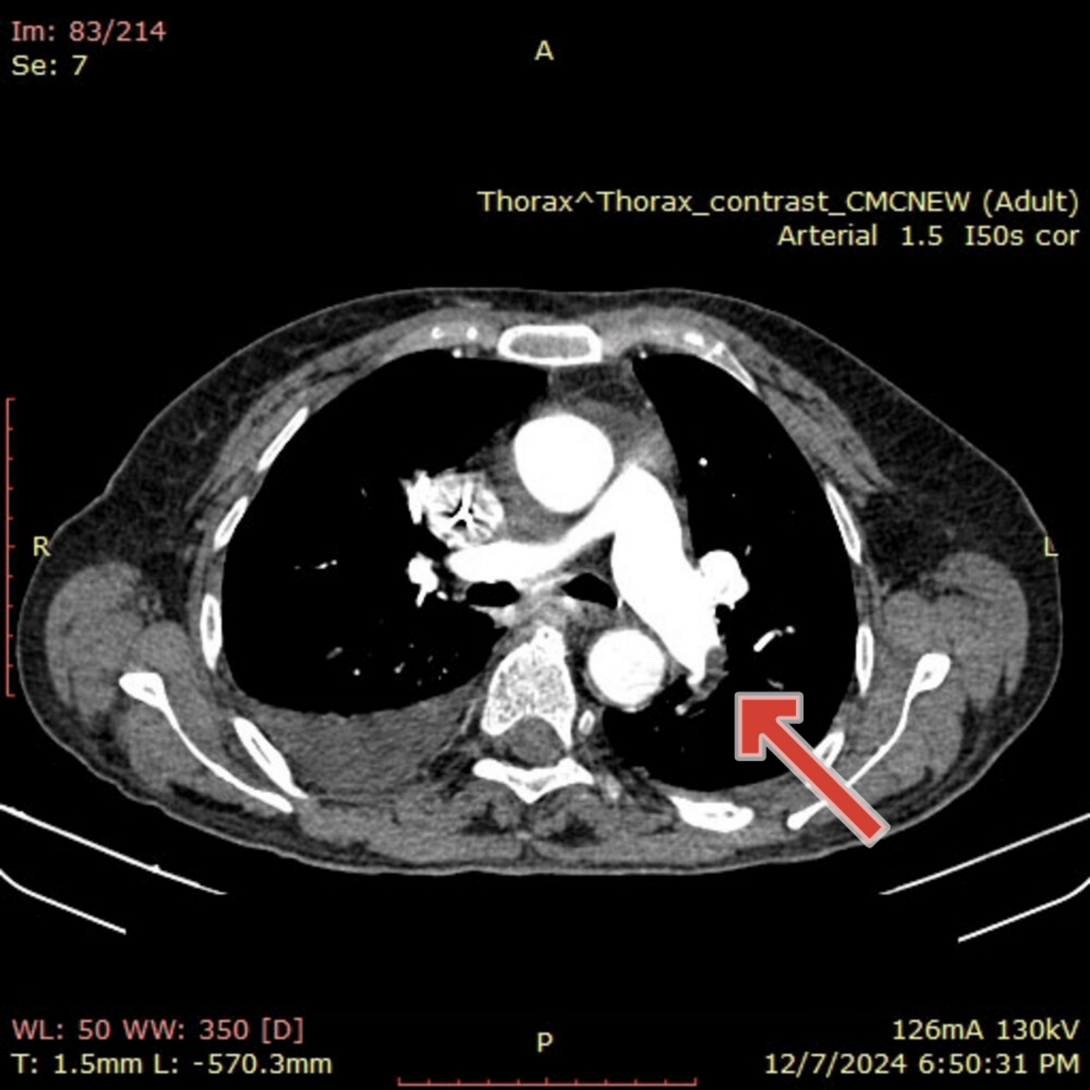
CT pulmonary angiography demonstrating pulmonary embolism CT pulmonary angiography revealed a central filling defect within the right pulmonary artery, consistent with an acute pulmonary embolism. The embolus appears as a sharply demarcated hypoattenuating area within the contrast-enhanced vessel, confirming the presence of an obstructive thrombus. The red arrow highlights this filling defect, which is located in a segmental or lobar branch of the right pulmonary artery, based on anatomical orientation and distribution.
